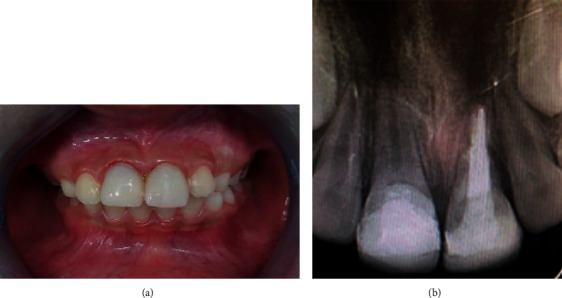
(a) Follow-up after 22 months. (b) Radiography after 22-month follow-up.
